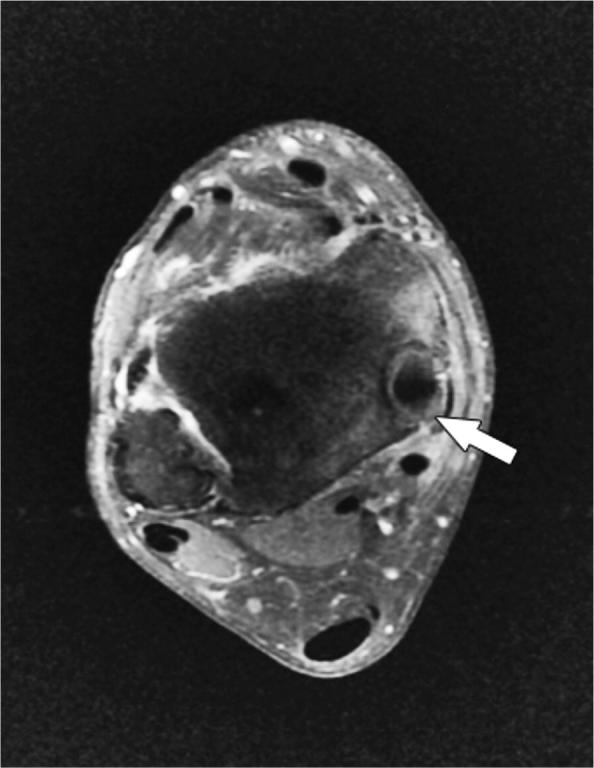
Tibialis posterior tendon tenosynovitis (arrow) on fat-saturation gadolinium injected T1-weighted axial MRI.
